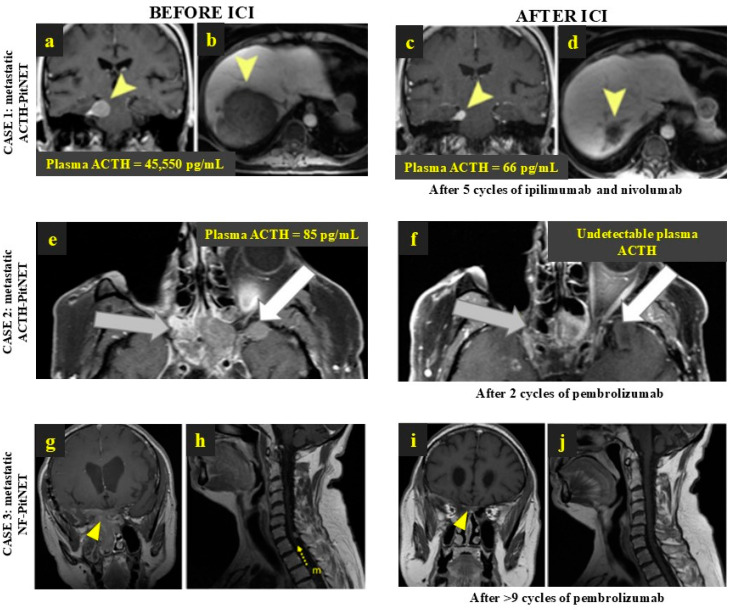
Remarkable response to anti-PD-1 treatment in 3 patients with metastatic PitNETs. CASE 1: Metastatic ACTH-PitNET with radiological and biochemical response to ipilimumab and nivolumab—brain and liver MRI before (a,b) and after ICI treatment (c,d). The yellow arrowheads point to the main tumour metastasis in the central nervous system and in the liver before and after ICI treatment. (Lin et al. 2018 [37], J Clin Endocrinol Metab (PMID: 30085142)). CASE 2: Metastatic ACTH-PitNET with radiological and biochemical response to pembrolizumab—MRI before (e) and after ICI treatment (f). The grey arrows point to tumour extension to the sphenoid sinus and posterior ethmoid air cells; white arrows indicate the tumour extension to the anterior and inferior aspects of the left temporal lobe. (Majd et al. 2020 [41], J Immunother Cancer (PMID: 33427689).) CASE 3: Metastatic NF-PitNET with radiological response to pembrolizumab, with >70% shrinkage in the main tumour and involution of spinal metastasis—MRI before (g,h) and after 1 year of ICI treatment (i,j). The yellow arrowheads show the primary PitNET before and after treatment, where a remarkable shrinkage of the tumour is visible; the yellow arrow with “m” letter depicts the spinal metastasis before ICI treatment, which involved following the treatment with pembrolizumab. (Feola et al. 2022 [11], Cancers (PMID: 36077631)). ACTH: adrenocorticotropic hormone; ACTH-PitNET: adrenocorticotropic hormone-secreting PitNET; FGFR: fibroblast growth factor receptor; ICI: immune checkpoint inhibitors; MRI: magnetic resonance imaging; NF-PitNET: non-functioning pituitary neuroendocrine tumour; PitNET: pituitary neuroendocrine tumour.
